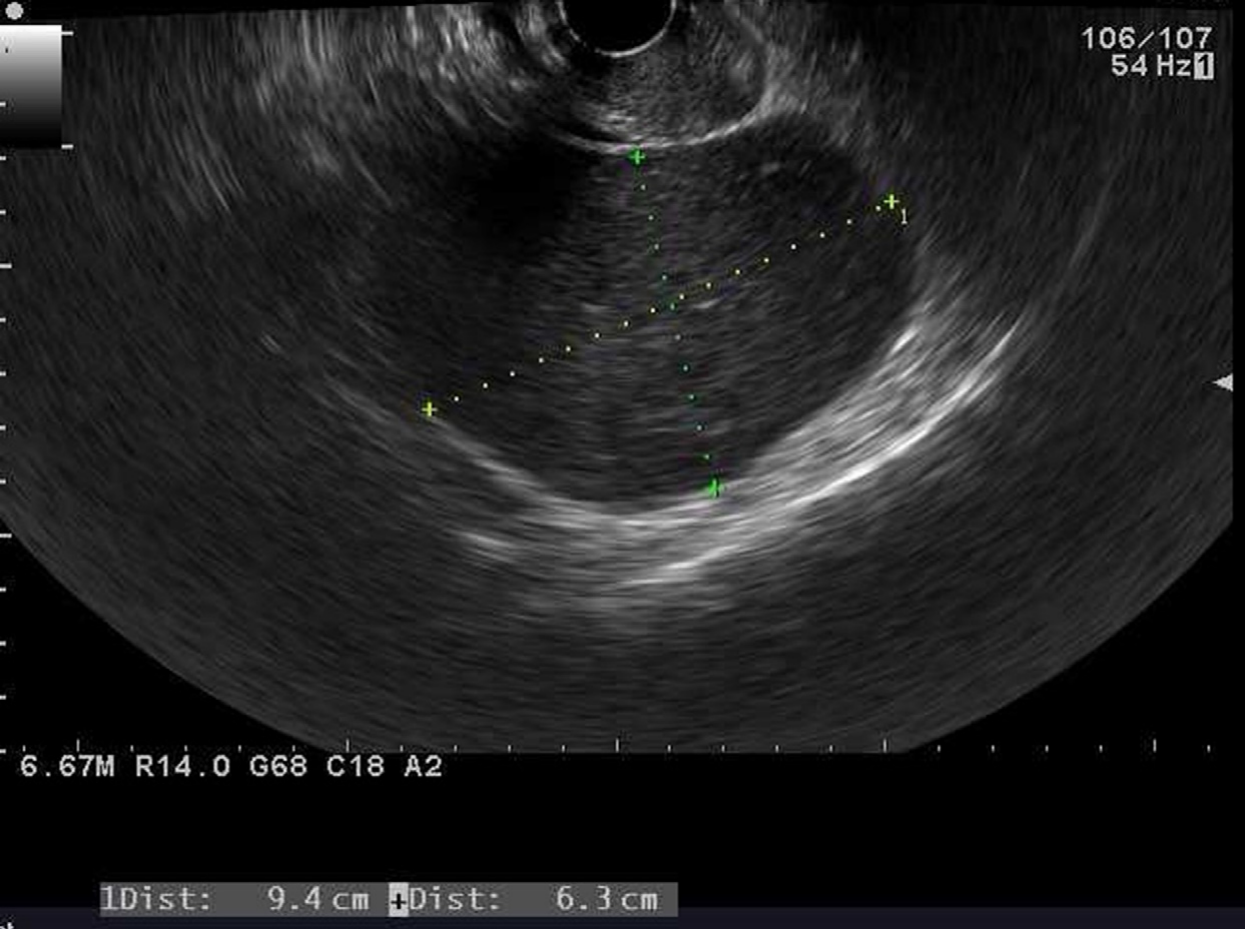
Transvaginal ultrasound image taken at the first visit that revealed a 9.4 × 6.3 cm ovarian endometrial cyst in Douglas' pouch.
